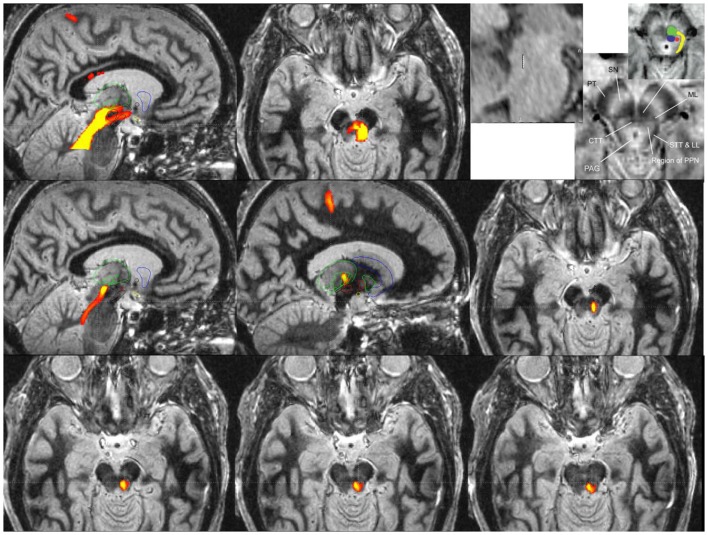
Probabilistic diffusion tractography for surgical targeting and planning of a PPN DBS implantation guided by an fast gray matter acquisition T1 inversion recovery (FGATIR) MRI scan.
